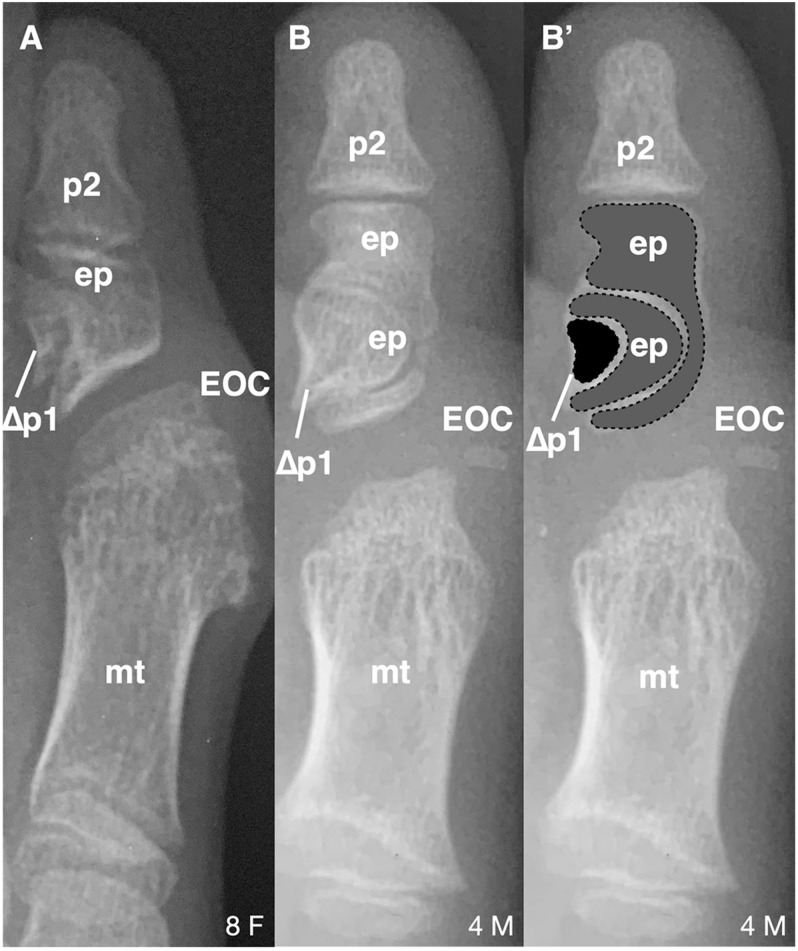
Longitudinal epiphyseal bracket in subjects with FOP. (A) Radiograph showing longitudinal epiphyseal bracket (LEPB; ep) of the proximal phalanx (p) of the great toe in a subject with FOP. A phalanx affected by LEPB may be referred to as delta phalanx, here labeled as Δp1. An apparent ectopic ossification center (EOC) distal to the metatarsal (mt) head is present. (B) Radiograph showing compound LEPB of the proximal phalanx, with outlines for clarity in (B’). Dotted lines denote distinct osseous elements occurring in concentric hemi-circles. Age (in years) and sex (F, M) of each subject, bottom right of each panel.
